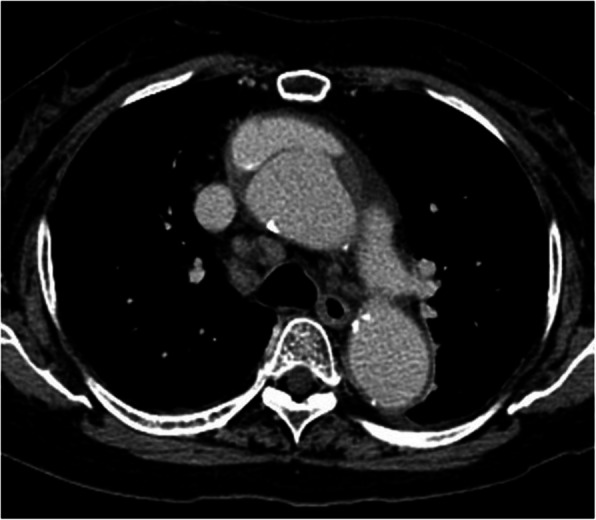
A computed tomography image before an urgent operation showing a patent false lumen at the ascending aorta
